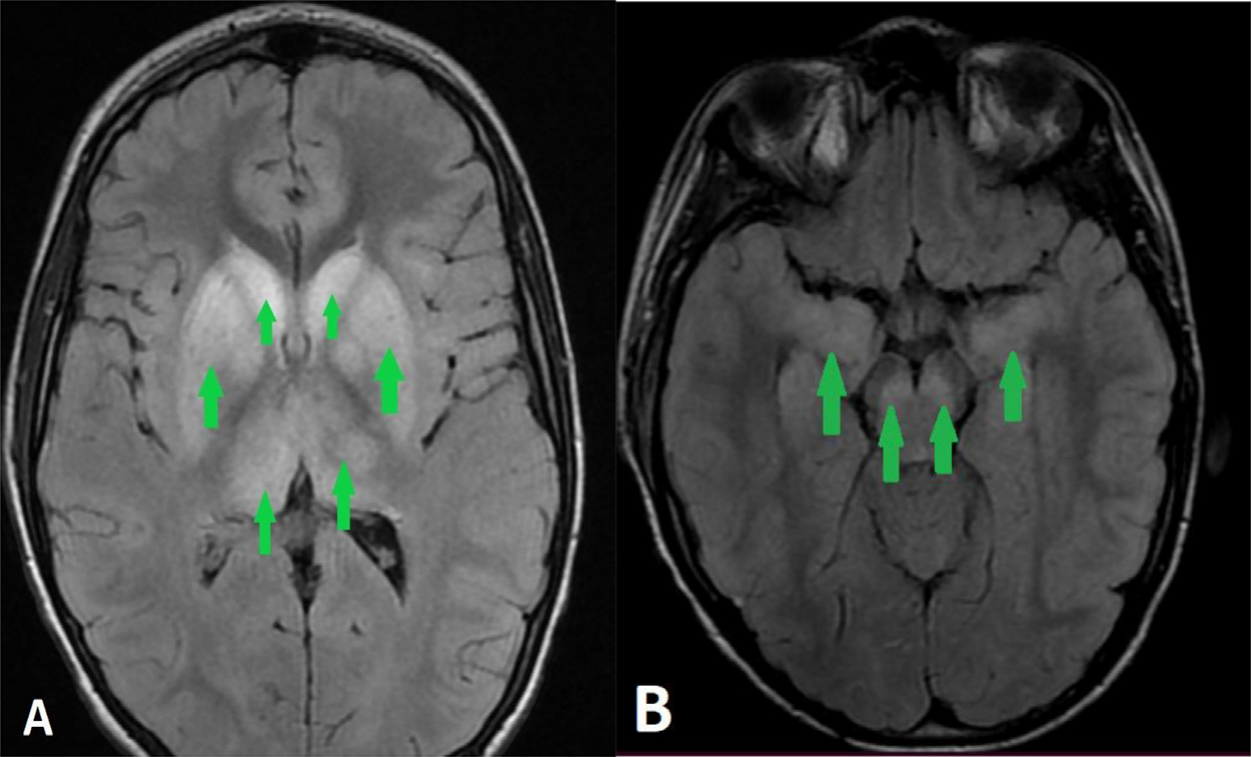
T2 FLAIR sequence showing a symmetrical hypersignal of basal ganglia (A), limbic cortex and substantia nigra (B) (green arrows).
